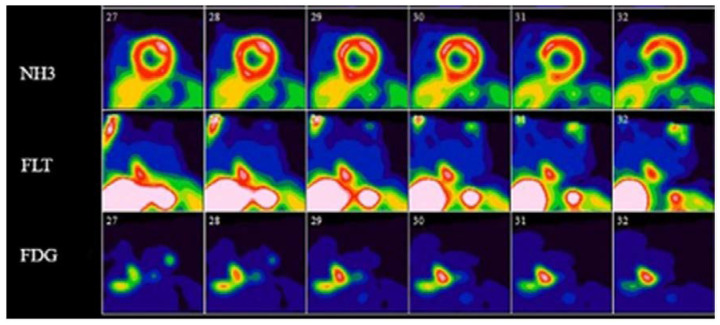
Short axis ^13^N-ammonia perfusion, ^18^F-FDG-, and ^18^F-FLT-PET images in a patient with active cardiac sarcoidosis. ^13^N-ammonia perfusion images (top row) show scarring of the inferior ventricular septum, with both FDG- and FLT-PET images showing evidence of active cardiac sarcoidosis in this area. Reprinted from ref. [75].
